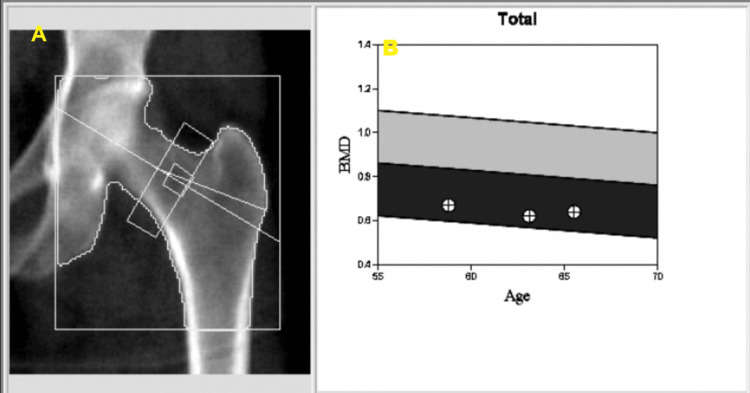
Evidence of osteoporosis affecting the left hip joint Panel A: The latest dual-energy X-ray absorptiometry (DEXA) scan study assessing the patient's left hip joint revealed evidence of osteoporosis. Left Hip bone mineral density (BMD) assessed as 0.638 g/cm2; T-score:-2.5; Z-score:-1.2; Classified as: Osteoporosis Panel B: The serial trend of low BMD results assessed in that joint is also presented as a graph.
